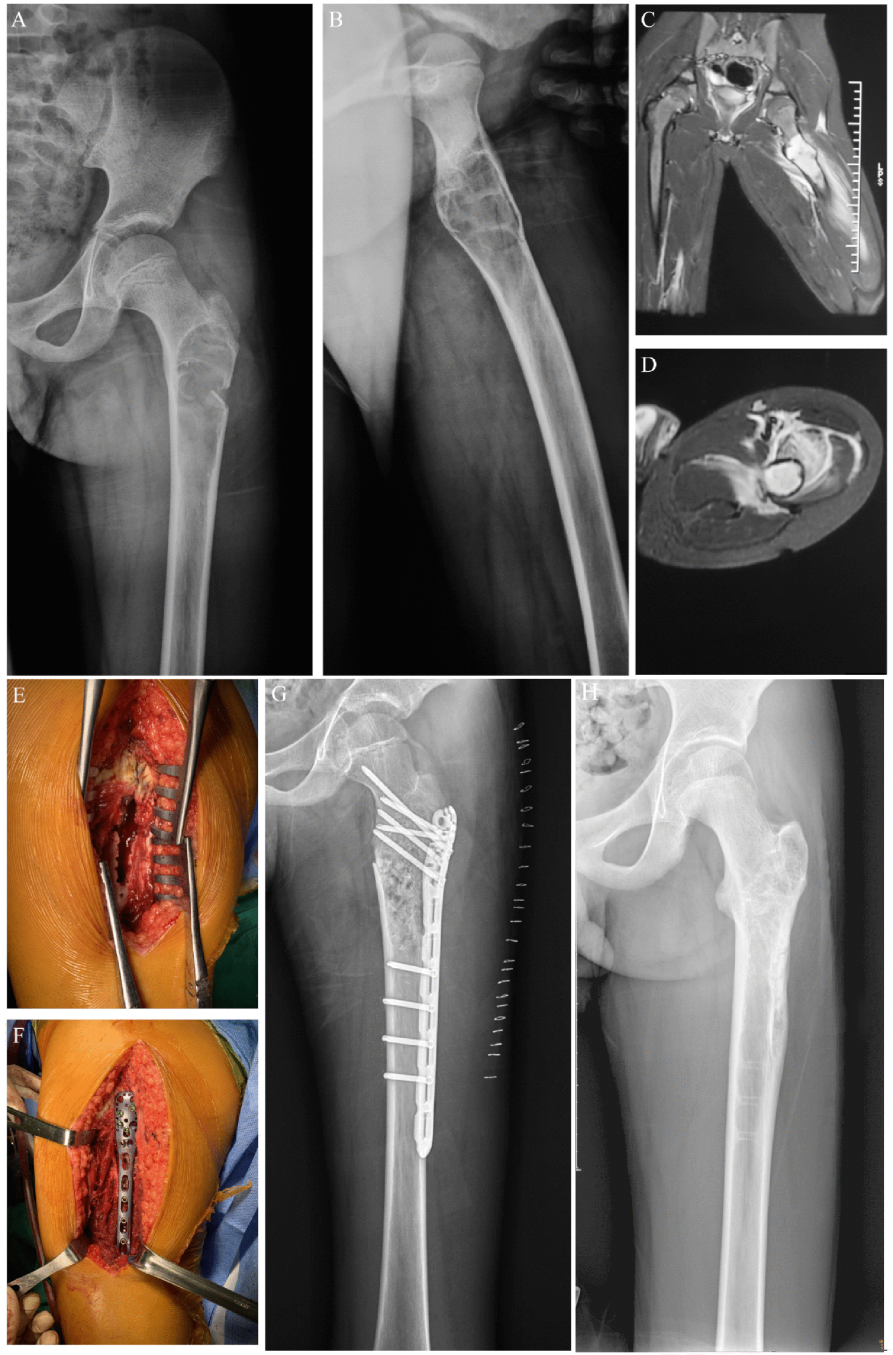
Preoperative, postoperative, and operative images of a pathological fracture of the proximal end of the left femur in an 11-year-old male patient. (A) Preoperative posterior radiographic image of the patient. (B) Preoperative lateral x-ray image of the patient. (C) Preoperative coronal MR image of the patient. (D) Preoperative transverse MR image of the patient. (E) Enlarged cortical window with adequate scraping of the lesion. (F) Bone grafting, internal fixation with a suitable adult 3.5 mm proximal humerus locking plate, and gross fracture reduction. (G) Postoperative x-ray image of the patient. (H) X-ray image of the patient after the removal of the metal plate.
